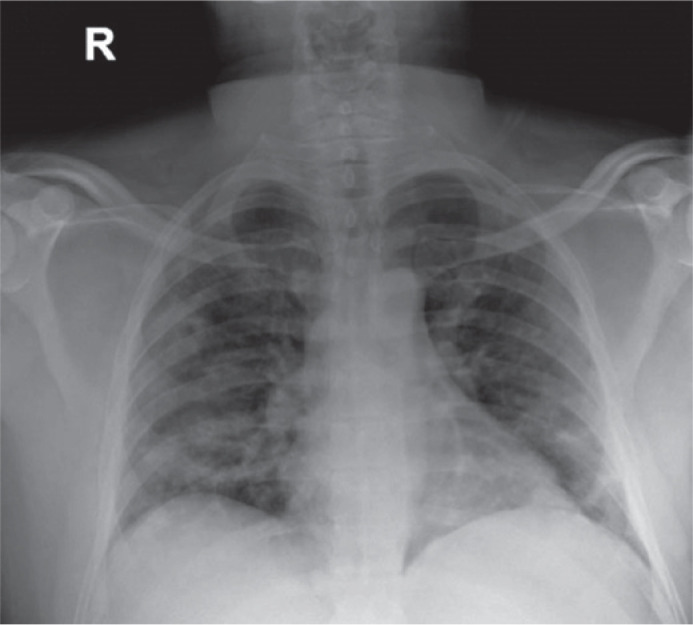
Chest radiograph of a patient with abducens nerve palsy and COVID-19.
